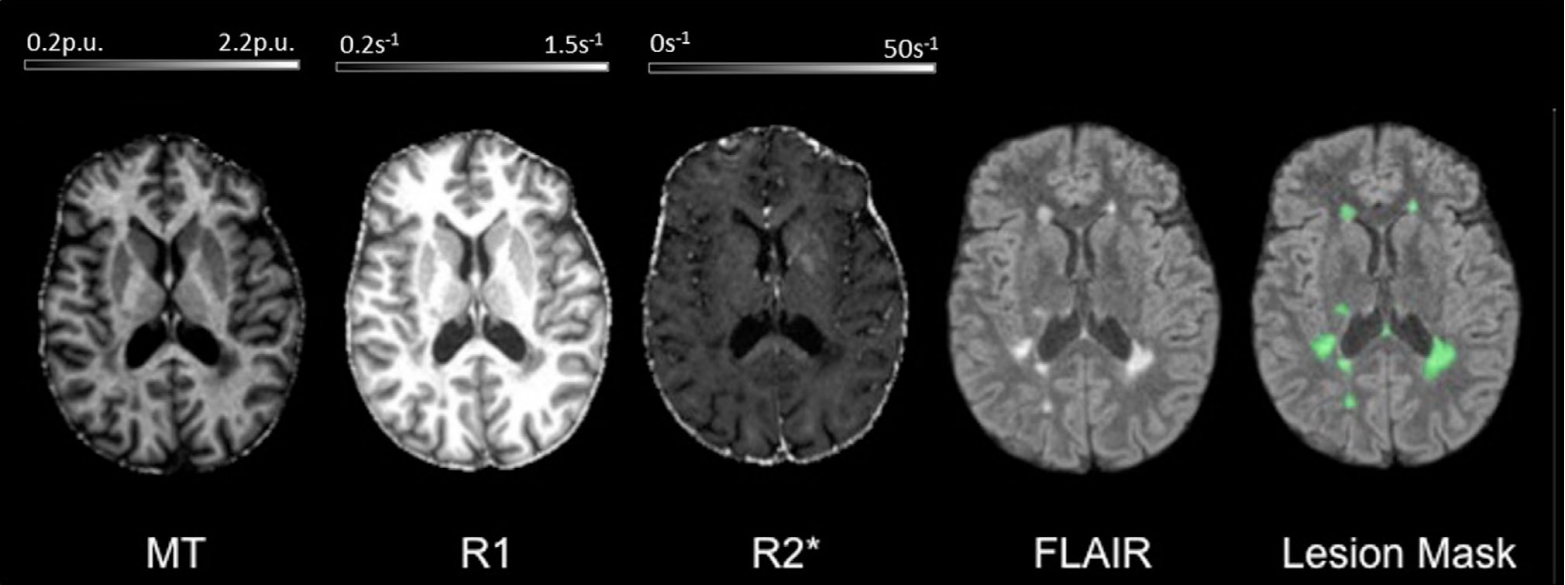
Example of MPM quantitative maps for a specific MS patient. From left to right: 3 MPM quantitative maps (MT, R1, R2*), standard FLAIR sequence image and FLAIR image overlaid with the estimated lesion mask. MT, magnetization transfer saturation; R1, longitudinal relaxation rate (1/T1); R2*, effective transverse relaxation rate (1/T2*); Lesion mask, posterior probability map of lesion tissue thresholded at 90%
